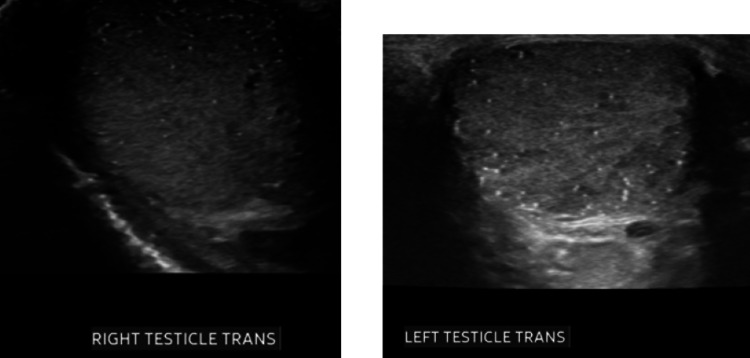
Testicular ultrasound. Right and left testicles showing echogenic small dots representing intratubular calcifications better known as testicular microlithiasis.
